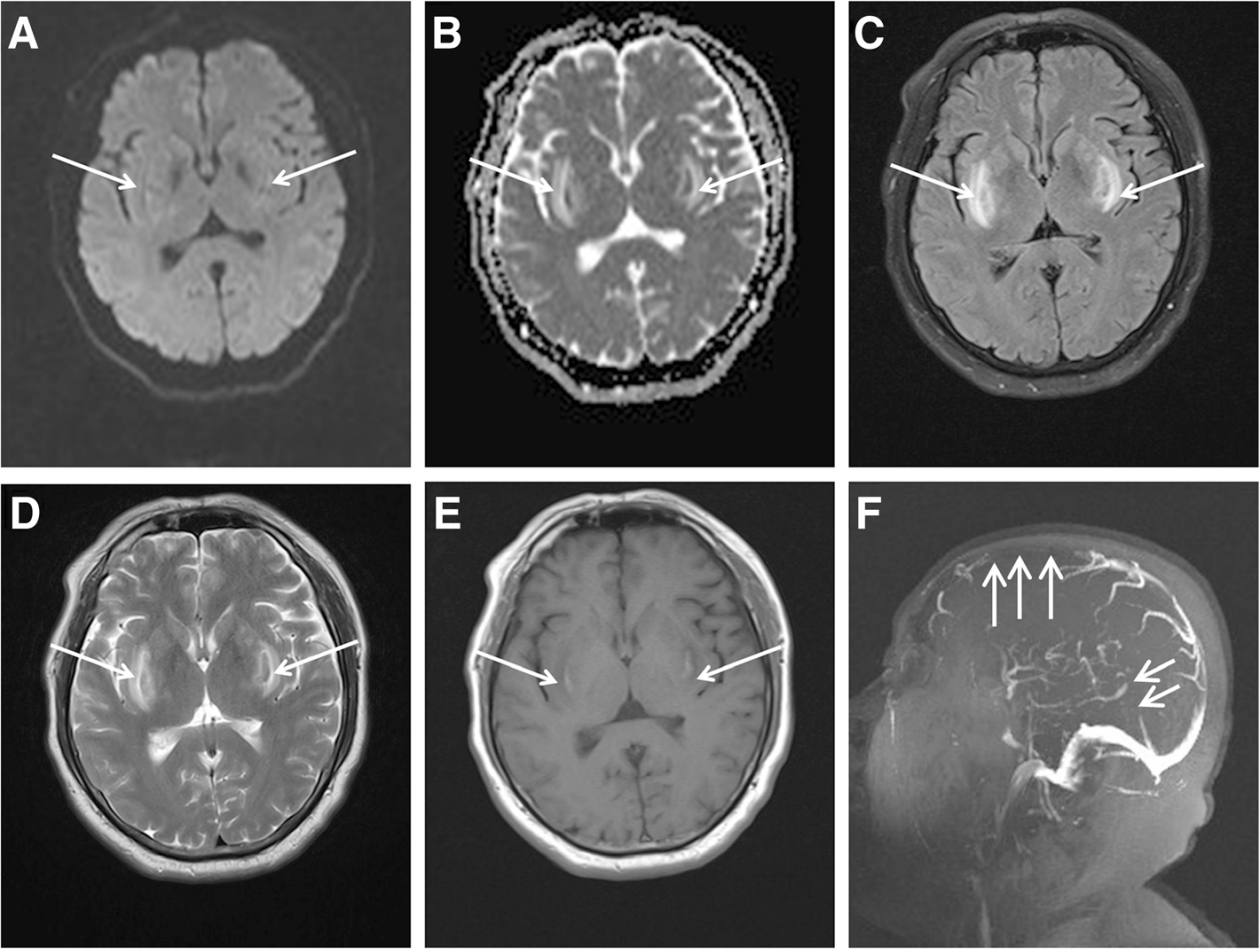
a-f. Brain magnetic resonance imaging (MRI) was performed 8 days after admission. Cerebral MRI revealed a slight hyperintense signal in the bilateral putamen on a diffusion-weighted imaging sequence and bilateral symmetrical hypointensity in the middle of the putamen as well as hyperintensity around hypointensity on b apparent diffusion coefficient, c fluid-attenuated inversion recovery, and d T2-weighted imaging sequence. The lesions were hyperintense in the middle of the bilateral putamen and hypointense around them on e T1-weighted imaging sequence. f The inferior sagittal sinus, straight sinus, and vein of Galen were not observed on magnetic resonance venography (MRV) performed on the 12th day of admission. The superior sagittal sinus was poorly developed based on MRV
